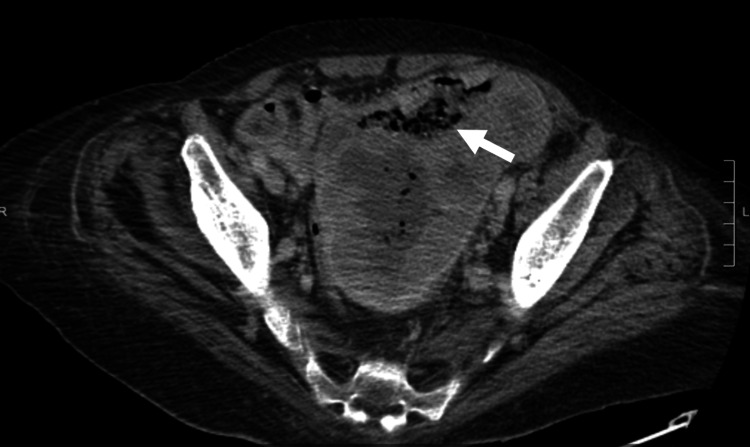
CT scan of the abdomen and pelvis showing complex air bubbles in the uterine cavity (white arrow) suggesting a fistulous tract between the uterine cavity and the small intestine.
